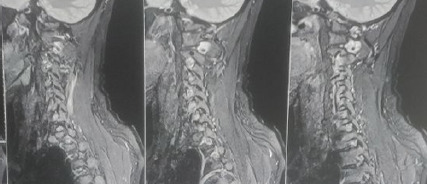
MRI showing high signaling intensity area involving the cervical spinal cord extending from cervicomedullary junction to C7 vertebrae
